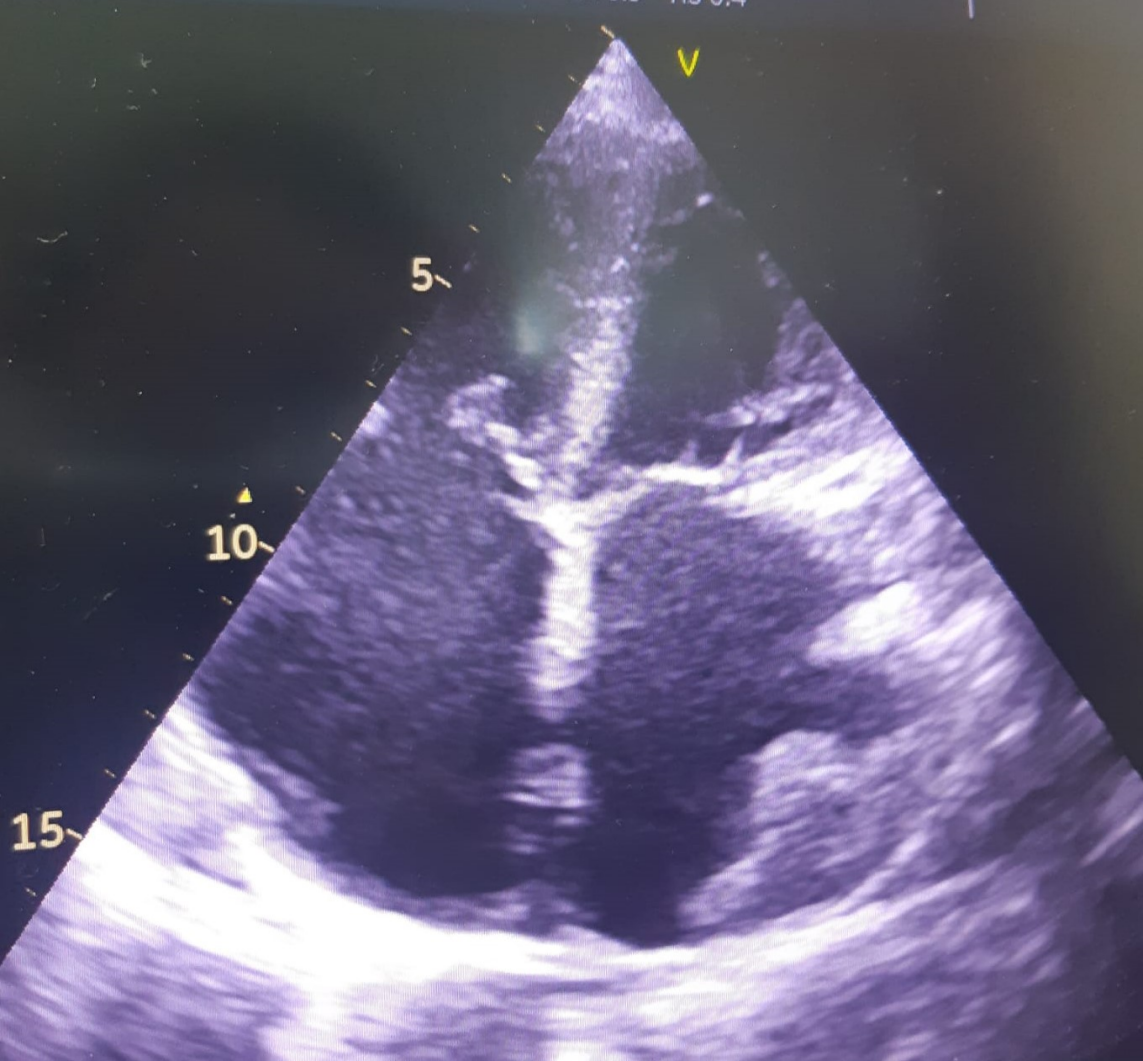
The echocardiographic aspect of RCM in an elderly patient
